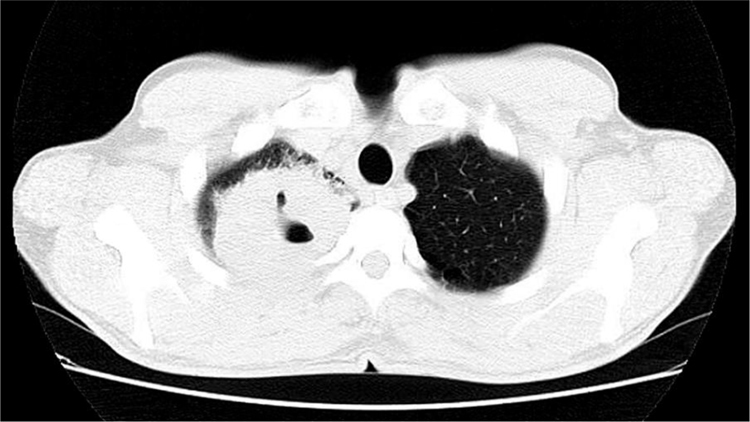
Computed tomography scan of the chest showing cavitation with a thick wall in the right upper lobe amid consolidations in other pulmonary areas.
